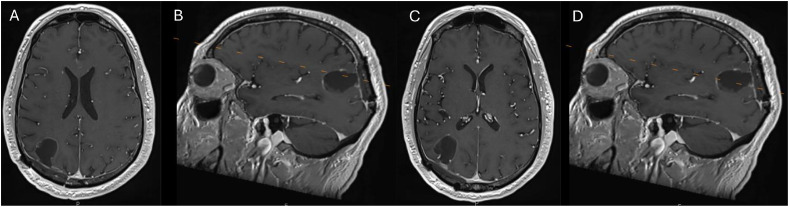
A magnetic resonance imaging (MRI) scan of the brain was performed 2 months after preoperative reirradiation and surgical resection. Postoperative changes are present in the right parietal lobe with improvement in surrounding edema and no evidence of progressive disease at treated sites or new brain metastases. (A) Axial MRI scan at the level of the superior aspect of the lesion. (B) Sagittal view with the orange dotted line denoting the level in (A). (C) Axial T1c+ at the level of the inferior aspect of the lesion. (D) Sagittal view with the orange dotted line denoting the level in (C).
